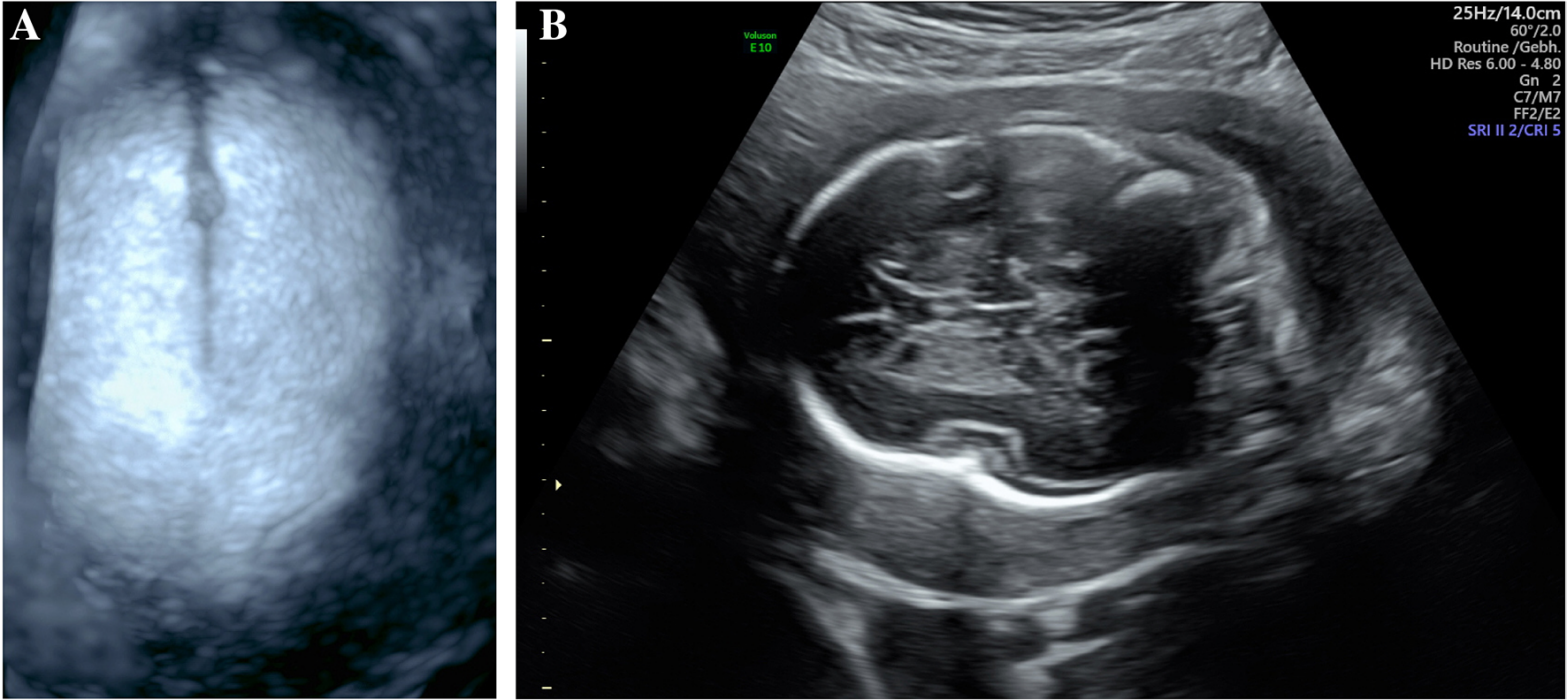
Fetus with partial sagittal craniosynostosis in 23 + 4 weeks of gestation. By three-dimensional ultrasonic skeletal imaging mode, a partial premature fusion of the sagittal suture can be shown (a). The B-mode image shows a prominent shape of the skull, a sagittal craniosynostosis is suspected (b)
